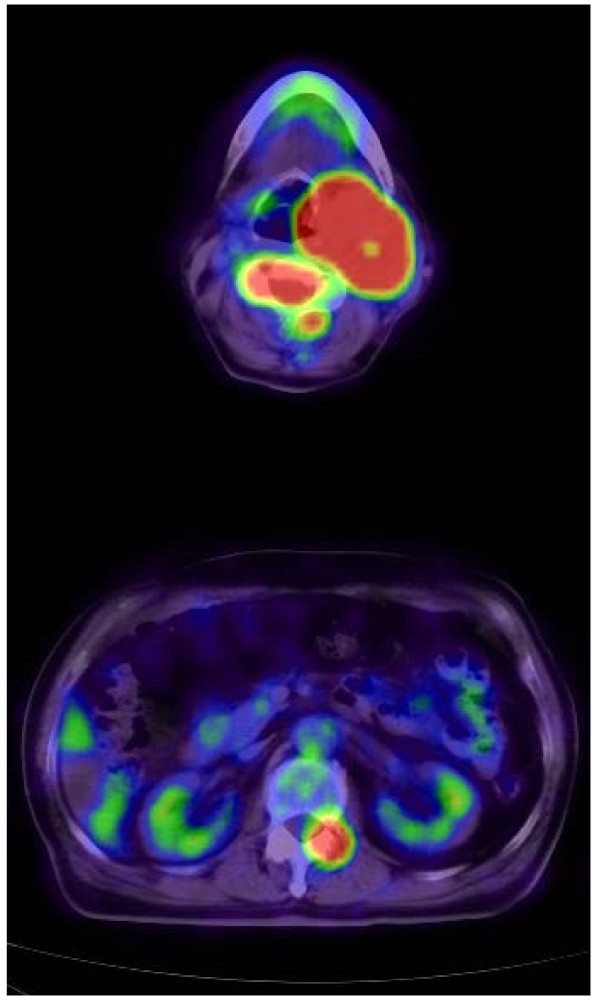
Positron emission tomography-computed tomography showing pathological fluorodeoxyglucose uptake in the neck (top) and in the C4 (top), L1 (bottom) vertebral bodies.
